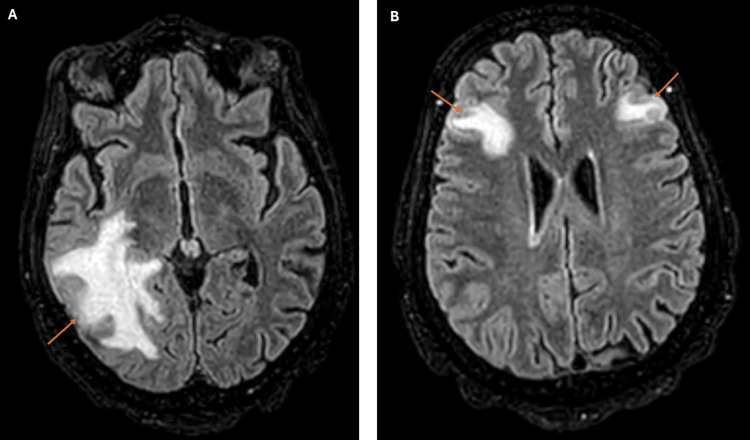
Brain MRI scan Two FLAIR images (A and B) of brain MRI showing areas of edema (arrows) in the right temporo-occipital (A) and bilateral frontal regions (B), causing sulcal effacement and locoregional mass effect. FLAIR: fluid-attenuated inversion recovery
